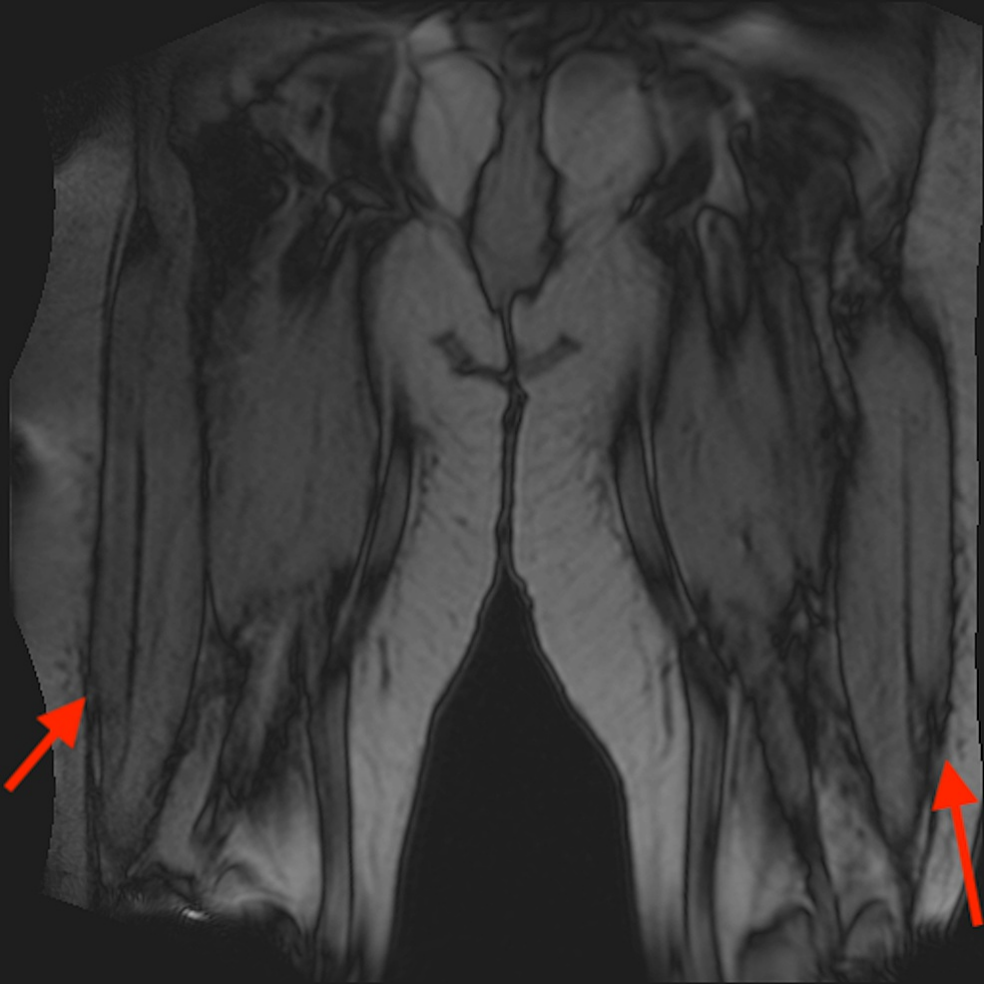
MRI coronal non-fat sat T1. No significant fatty atrophy of posterior thigh muscles.
